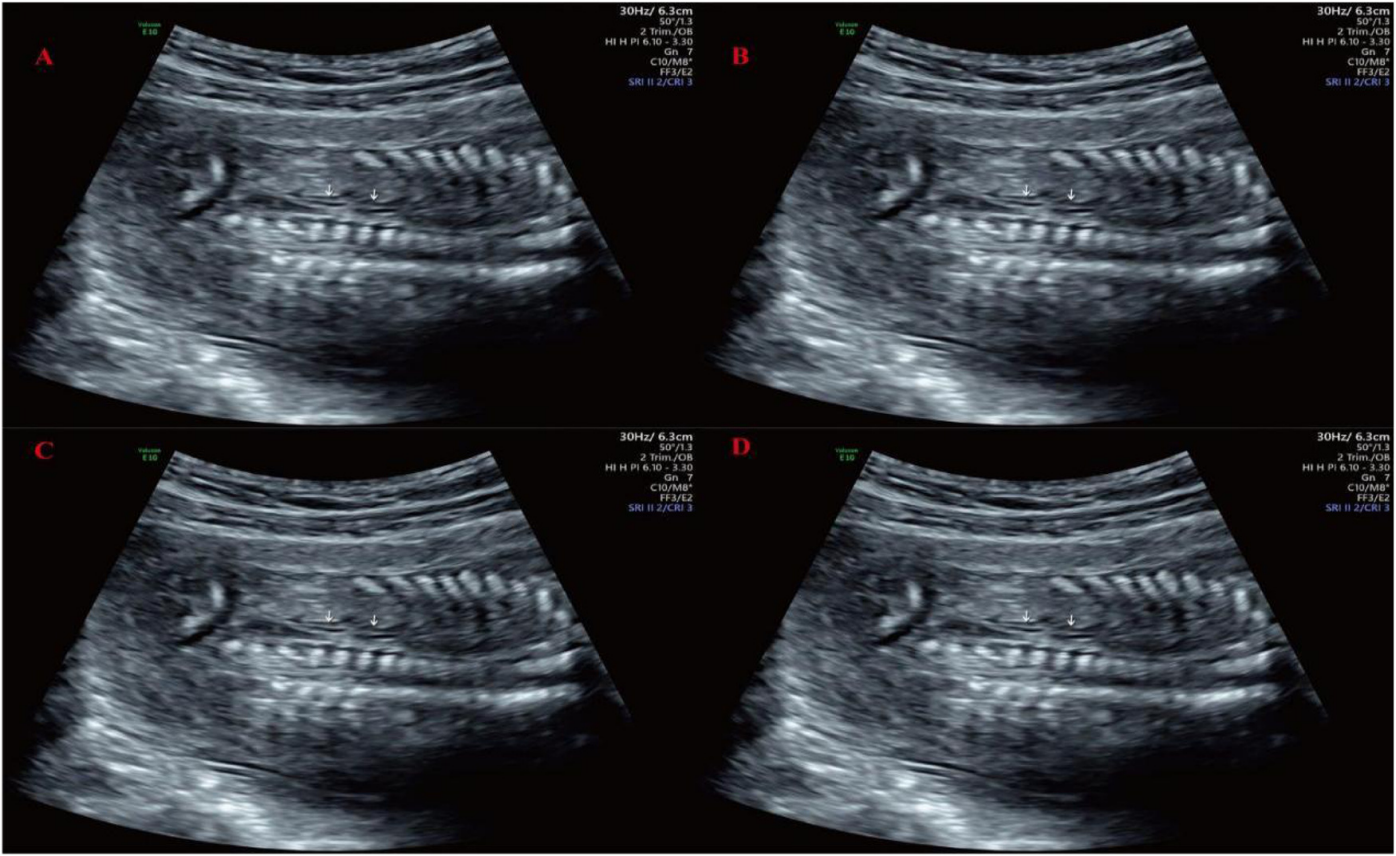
Prenatal ultrasound imaging at 17-week-6-day of gestation. (A) Adrenal gland lying-down sign; (B) Transverse section of the right kidney; (C) Coronal section of the right kidney; (D) Only the right renal artery was observed on Doppler imaging, with no identifiable course of the left renal artery.
